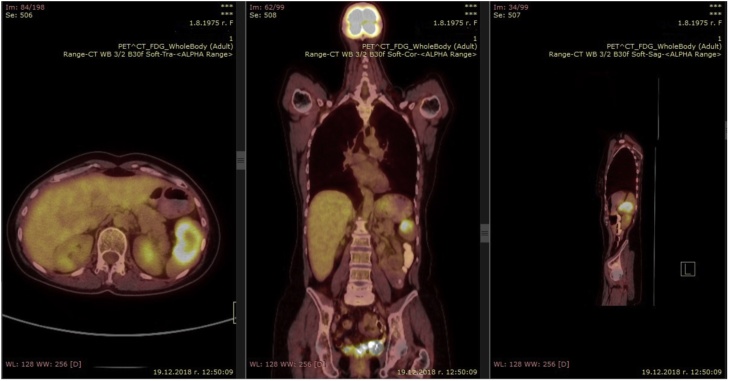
PET/CT of patient with spleen metastasis from rectal cancer – case 2.
